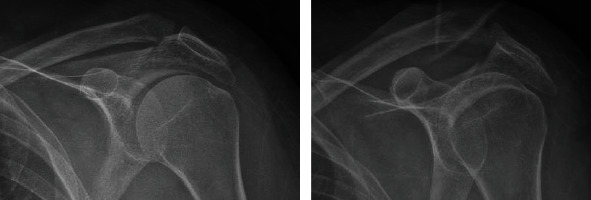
Anteroposterior X-ray imaging showing an acromion fracture and concomitant distal clavicle fracture.
